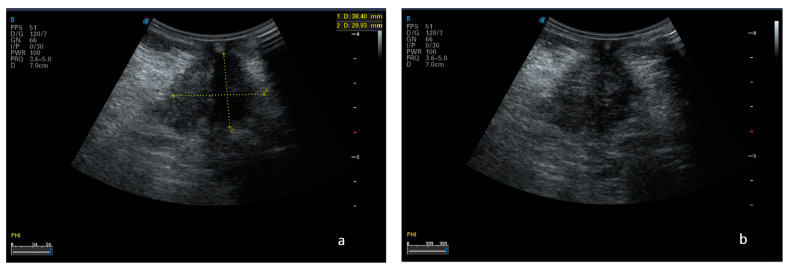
Ultrasound scan (a,b) of an 81-year-old man’s abdomen in supine position reveals a 3.8 × 2.9-cm hypoechoic lesion (nodule of peritoneal carcinosis) on the middle abdominal wall.
